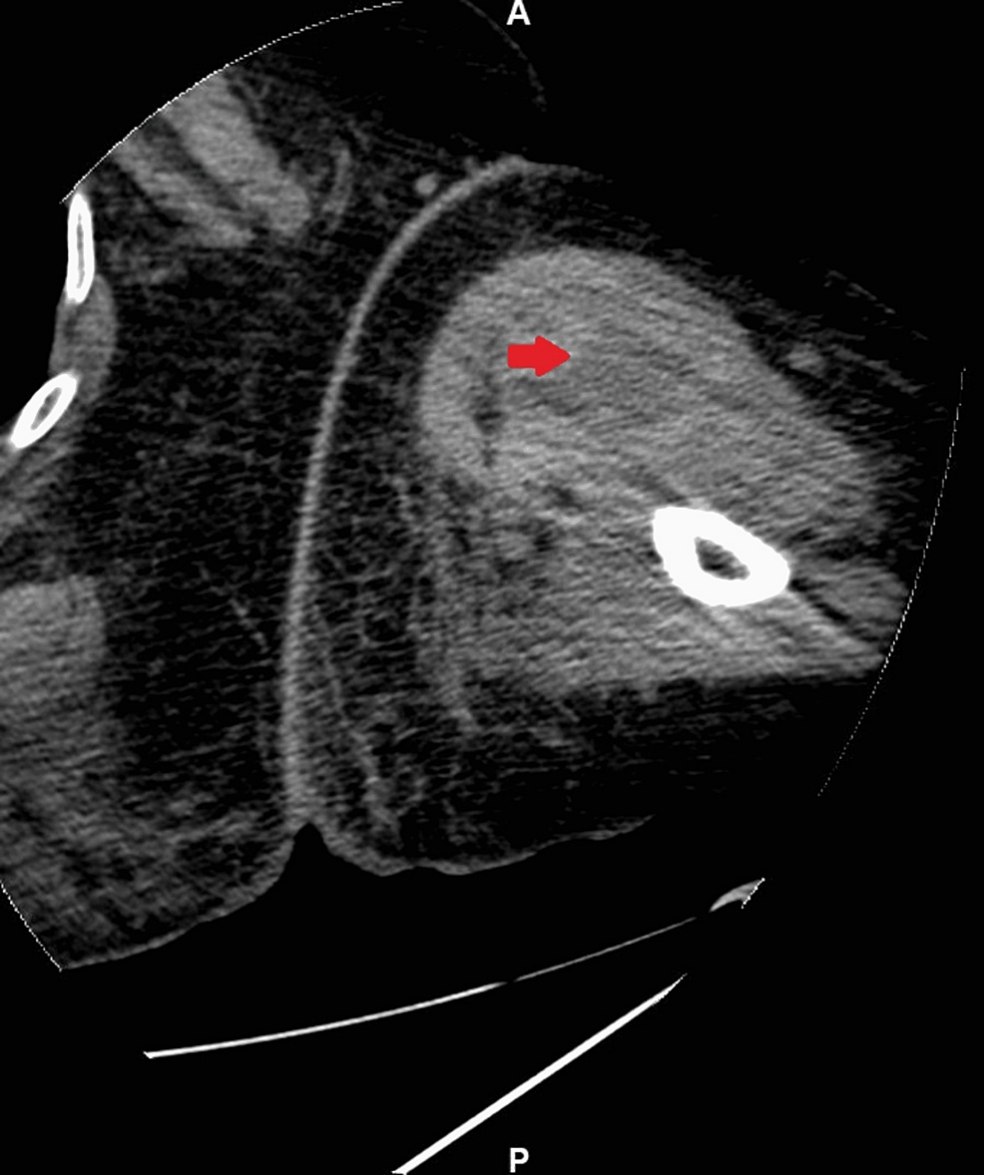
CT of the left upper extremity without contrast showing a 4 × 6 × 10 cm hypoattenuating mass in the biceps muscles showing possible hematoma.
